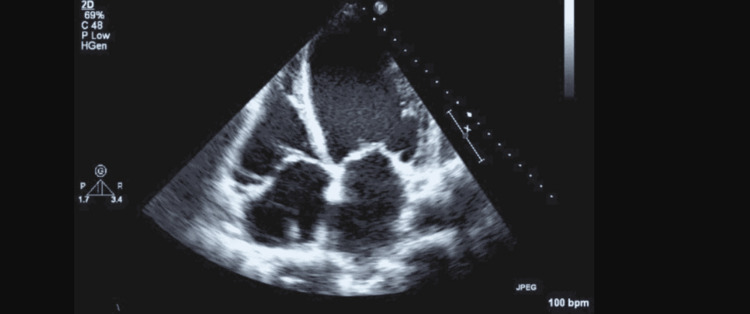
Case 1: Apical four-chamber echocardiographic view demonstrating dilated cardiomyopathy with left ventricular dilation and an ejection fraction of 40-45%.
